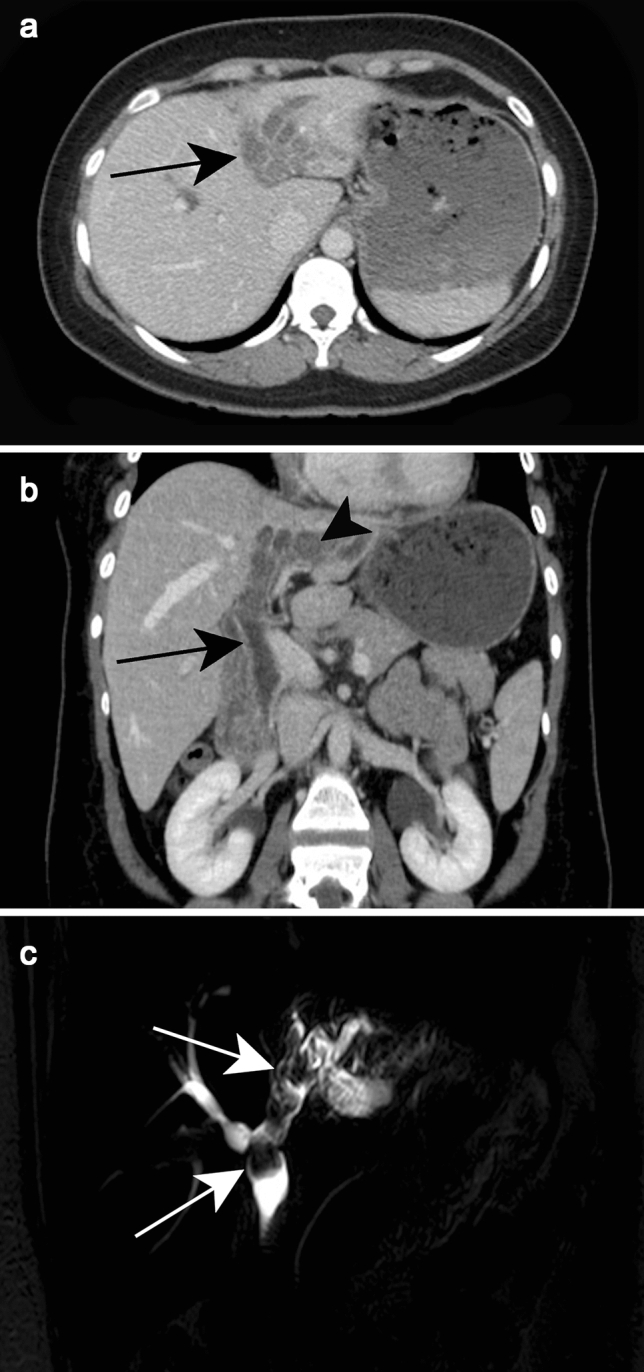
Ascariasis in a 36-year-old male patient. Axial and coronal reformatted contrast-enhanced CT (a, b) show intrahepatic and extrahepatic bile duct dilatation (black arrows). Note intrahepatic bile ducts filled with structures more attenuating than bile (black arrowhead), indicating adult worms. Oblique coronal single-shot fast spin-echo MR cholangiogram (c) shows adult worms as serpiginous and nodular filling defects in the left intrahepatic and extrahepatic bile ducts (white arrows)
